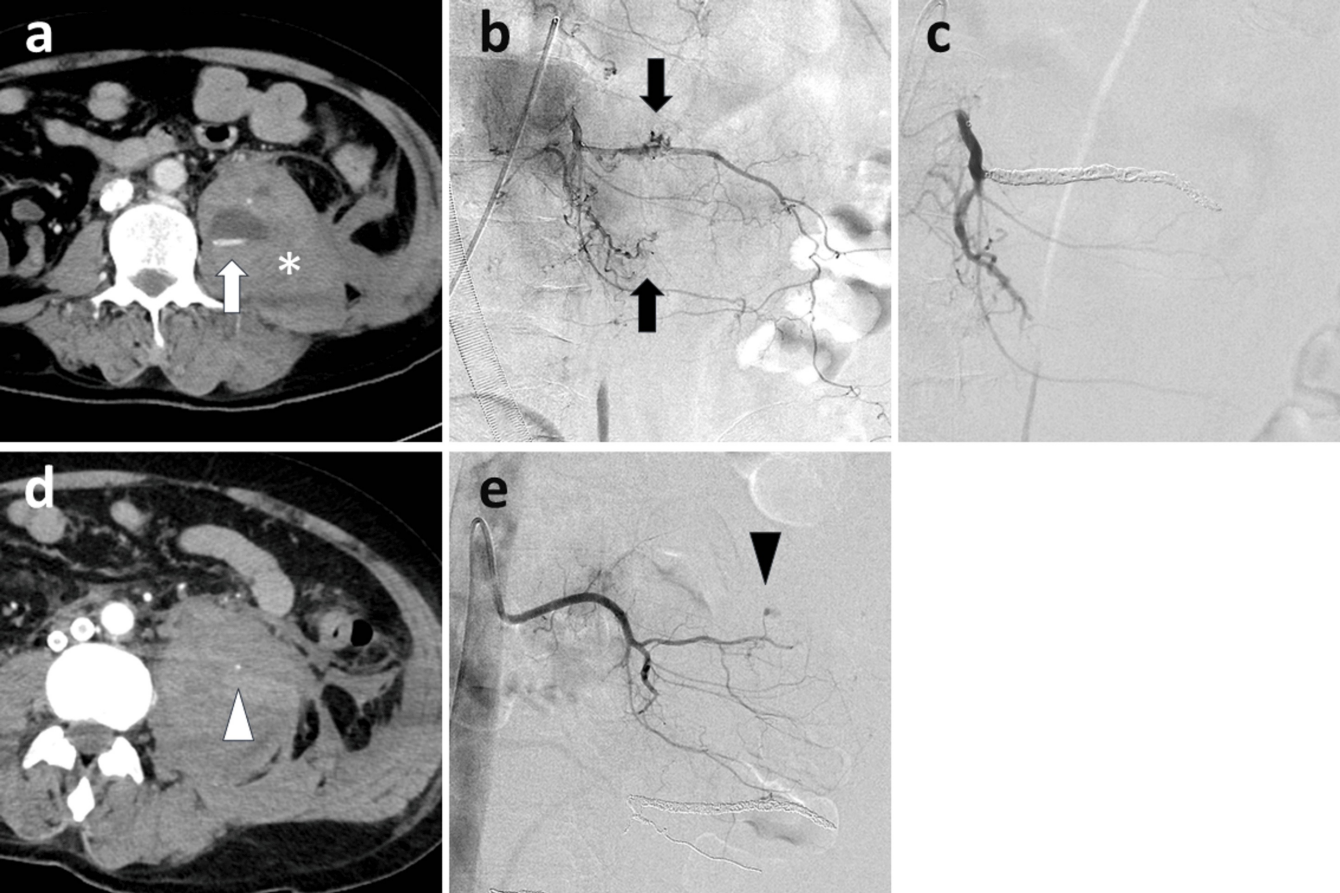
Imaging of a 50-year-old man who underwent extracorporeal membrane oxygenation owing to a severe coronavirus disease infection a: CECT reveals a hematoma (*) and contrast extravasation (white arrow) in the left psoas muscle. b: Left second lumbar arteriography demonstrates multiple sites of contrast medium extravasation (arrow). c: The second lumber artery is subsequently embolized using a coil and gelatine sponge. d: Two days after the initial transcatheter arterial embolization, CECT shows rebleeding (white arrowhead) in a region of the left psoas muscle that is a distinct area from the prior bleeding. e: Angiography of the first left lumbar artery shows new contrast medium extravasation at multiple sites (arrowhead), which was subsequently embolized using a gelatine sponge. CECT: contrast-enhanced computed tomography
